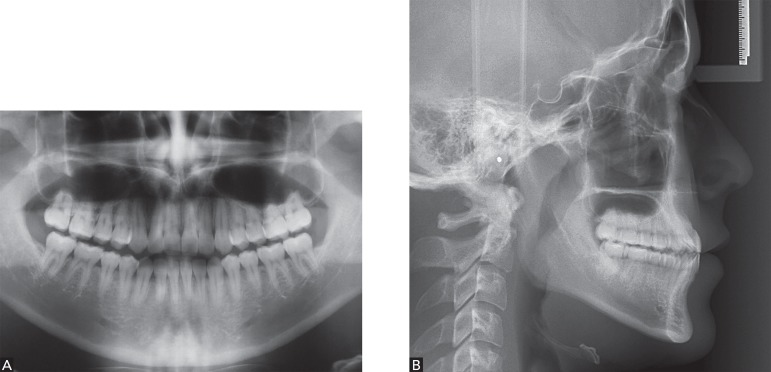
Initial radiographs: A) Panoramic radiograph; B) Profile radiograph.
